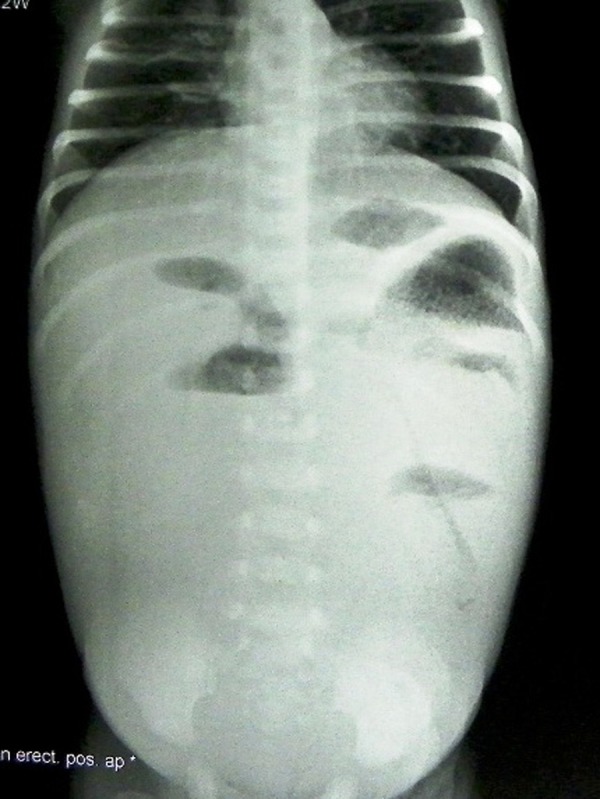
Figure 1: Plain X-ray abdomen shows distal bowel obstruction.
